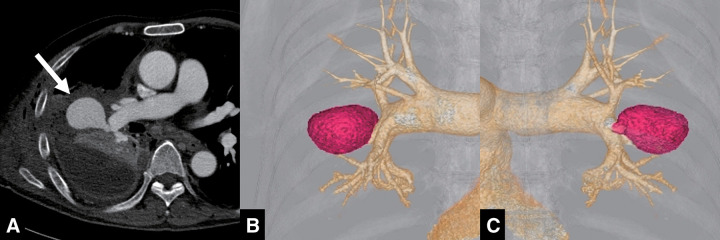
CT pulmonary angiography before embolization. (A) The pseudoaneurysm (white arrow), surrounded by empyema, extended from the interlobar segment of the right pulmonary artery. (B) Three-dimensional angiographic reconstruction (anterior–posterior view) revealed the pseudoaneurysm (red region) arising from the interlobar segment of the right pulmonary artery. (C) Three-dimensional angiographic reconstruction (posterior–anterior view). CT: computed tomography
